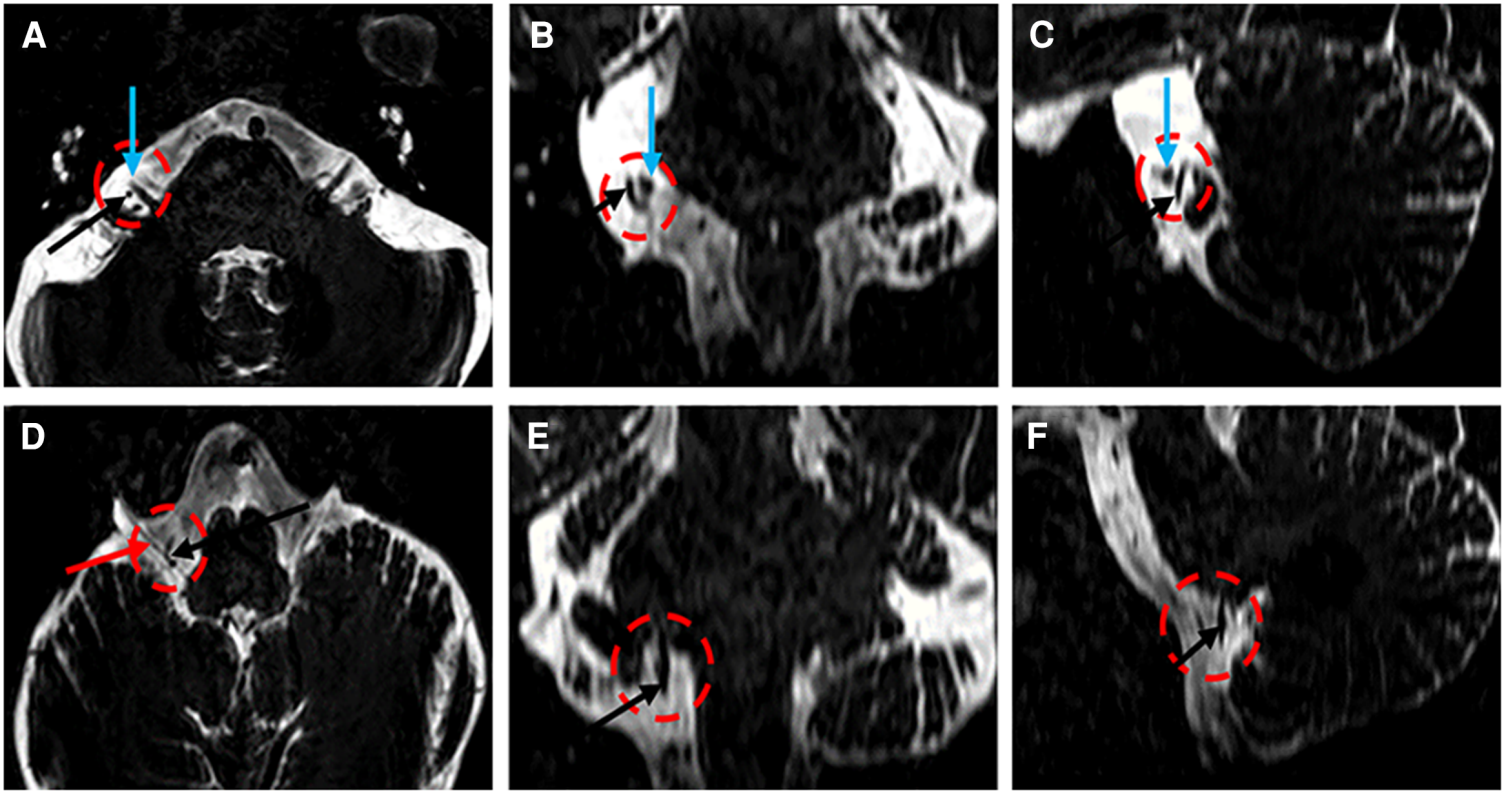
Three-dimensional T2-weighted images (A–F) demonstrating the AICA (A–F, black arrow), the VII/VIII complex (A–C, green arrow), and the vagus nerve (D, black arrow).
